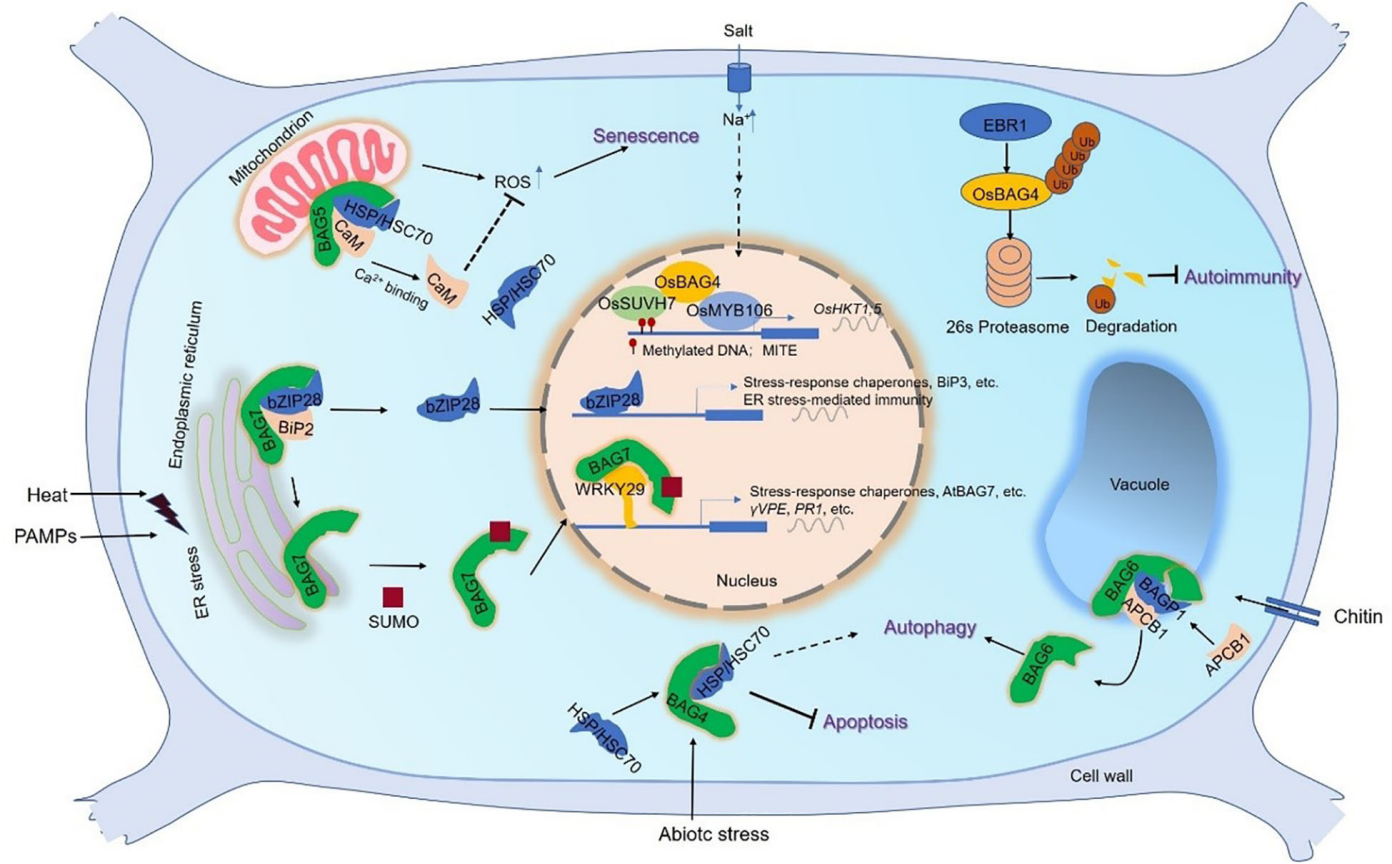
The working model of plant BAG-mediated signal pathway. The roles of BAG1–3 are less known. BAG4 plays a role in the cytoplasm, combines with HSP70/HSC70 chaperones, and is involved in the inhibition of cell death under abiotic stress (Hoang et al., 2015). Under salt stress, the rice OsBAG4 acts as a bridge between OsSUVH7 and OsMYB106 to promote the binding of OsMYB106 to MYB binding MYBE of OsHKT1;5 promoter, thereby activating *OsHKT1;5* expression to decreased salt sensitivity. OsSUVH7 reads the methylation of the MITE of OsHKT1;5 promoter (Wang et al., 2020a). The rice RING-type E3 ubiquitin ligase, EBR1, directly targets OsBAG4 for ubiquitination-mediated degradation (You et al., 2016). The mitochondrion-localized AtBAG5 forms a complex with CaM/HSC70 to regulate plant senescence. The complex promotes the production of ROS and accelerates leaf senescence at low Ca^2+^ levels. In contrast, the senescence is inhibited at high Ca^2+^ levels (Li et al., 2016a). BAG6 is involved in autophagy and is part of a fundamental defense pathway against necrotizing fungi. BAG6 interacts with BAGP1 and APCB1, both of which are necessary for BAG6 processing. BAG6 cleavage triggers autophagy in the host which (Li et al., 2016b). BAG7 mediated heat stress tolerance (Li et al., 2017). In ER, under normal conditions AtBAG7 binds AtBiP2 and AtbZIP28 transcription factors. Under heat stress conditions, unfolded proteins accumulate and AtBAG7 and AtbZIP28 dissociate from AtBiP2. Then AtBAG7 is sumoylated and proteolytically cleaved to translocate to the nucleus. Here, AtBAG7 interacts with WRKY29, which is able to induce the transcription of *AtBAG7* and other chaperone genes. Besides, AtbZIP28 enters the nucleus to induce the chaperone gene expression including AtBiP3. Similar to heat stress, ER stress is triggered when Phytophthora PAMPs are infected (Zhou et al., 2021). BAG7 binds to WRKY29 to regulate plant immunity mediated by ER stress, which is also directly activated by bZIP28. Thus, plants exhibit resistance to Phytophthora infection. Abbreviations: BAG, Bcl-2-associated athanogene; BAGP1, BAG-associated GRAM protein 1; BIP2, Binding immunoglobulin protein 2; bZIP28, basic leucine zipper 28; CaM, calmodulin; ER, endoplasmic reticulum; HSC70/HSP70, heat shock cognate 70/heat shock protein 70; MITE, miniature inverted repeat transposable element; ROS, reactive oxygen species; SUMO, small ubiquitin-like modifier; WRKY29, WRKY DNA-binding protein 29.
